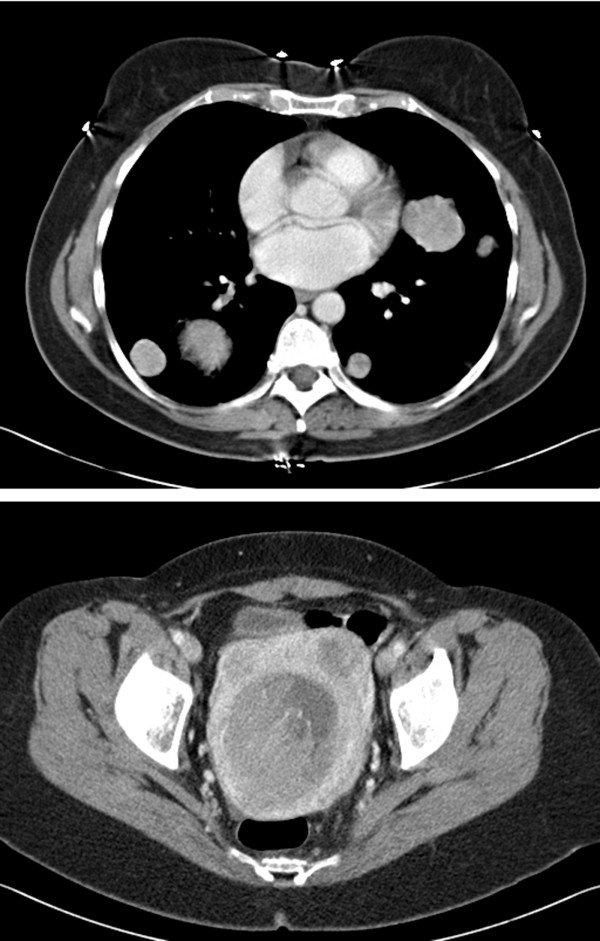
CT scan at start of chemotherapy.
